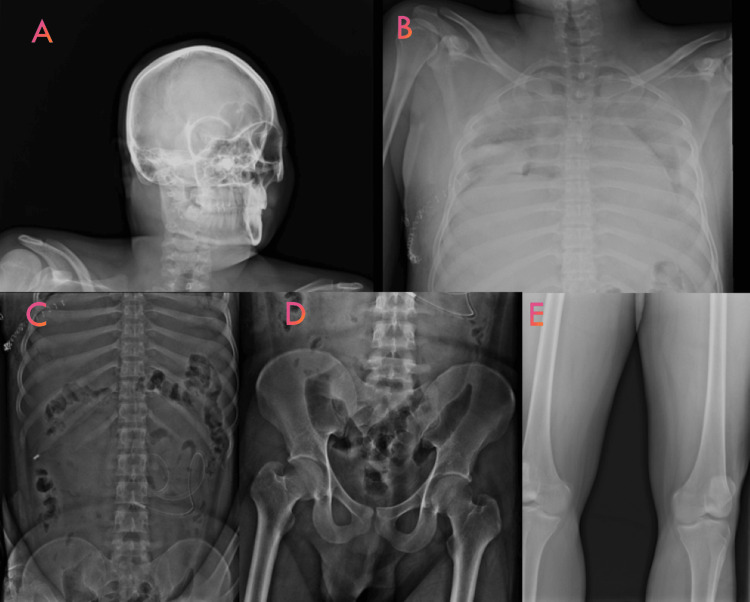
X-ray of the entire body from head to toe (A: head, B: neck and chest cavity, C: abdominal cavity, D: pelvic cavity, and E: lower limb) performed prior to the autopsy shows no evidence of a bullet
